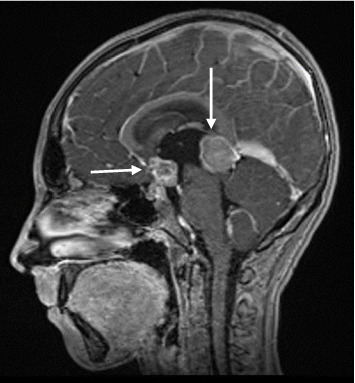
Sagittal T1 contrast-enhanced magnetic resonance images showing suprasellar and pineal lesions with heterogeneous enhancement. Moderate obstructive hydrocephalus was visible on additional views.
